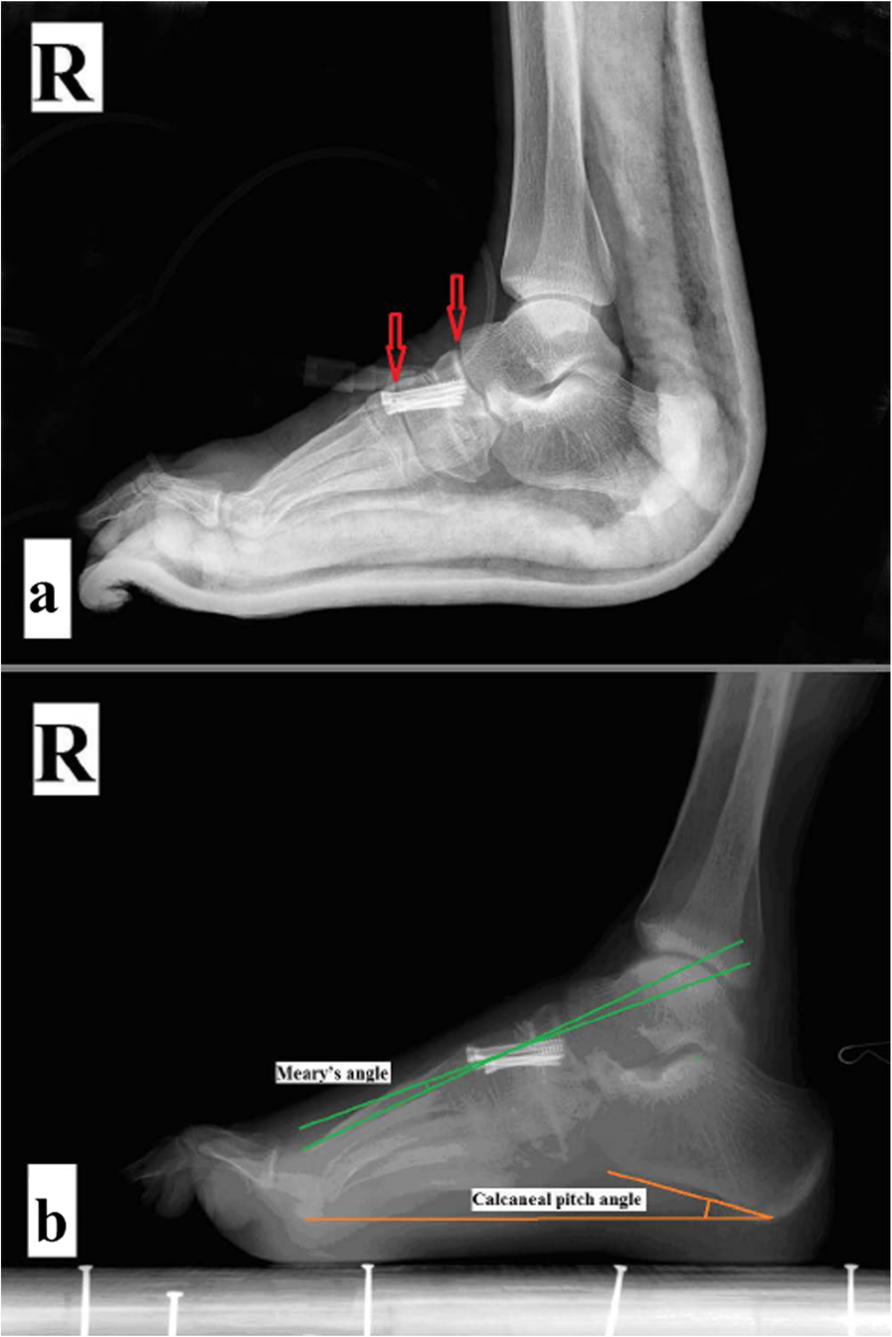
Postoperative X-ray. (a) The adjacent joint sparing internal fixation between the Lisfranc line and the Cyma line (arrow) is shown. (b) Bony union of the midfoot osteotomy and no obvious degeneration of adjacent joints 6 months postoperatively is shown.
